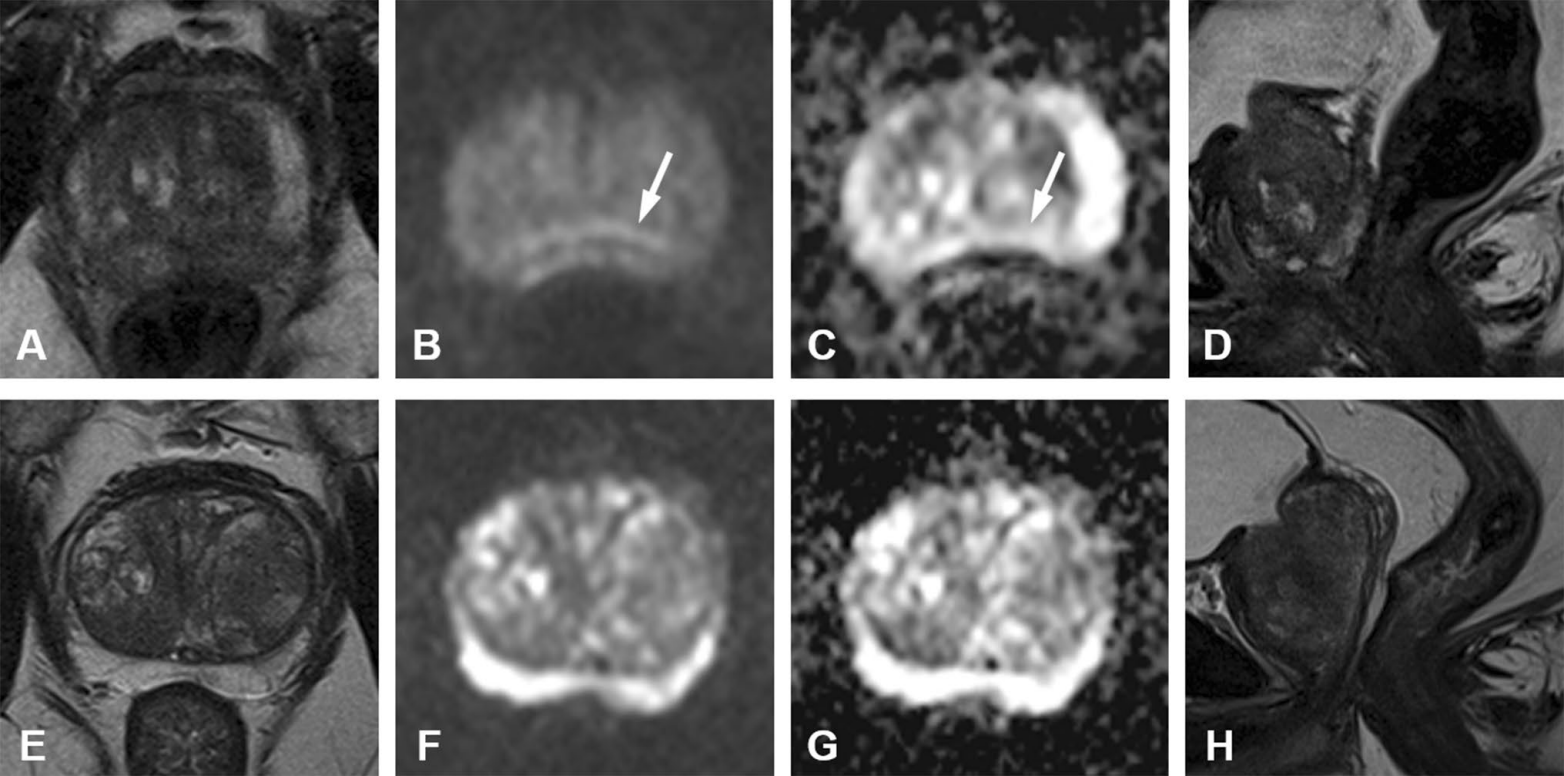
Multiparametric MRI of the prostate of a 70-year old patient (P1) within the cohort HBB−/ME−/DR− (a–d) compared to a 55-year old patient (P2) within the cohort HBB−/ME+/DR− (e–h). P2 applied microenema (ME) before MRI scan, P1 did not. Both patients did not receive hyoscine *N*-butylbromide (HBB). A transverse and sagittal T2w, a DWI b-1000 and the corresponding ADC map are shown. Note the presence of susceptibility artifacts (white arrows) on the posterior border of the prostate on the DWI b-1000 and ADC map in P1 without ME (b, c), which presumably explained due to an increased amount stool/gas in the rectum, particularly well visualized on the sagittal T2w (d), as compared to P2 (h). Also note the relatively increased blurring on the T2w of P1 (a), as compared to the P2 (e)
